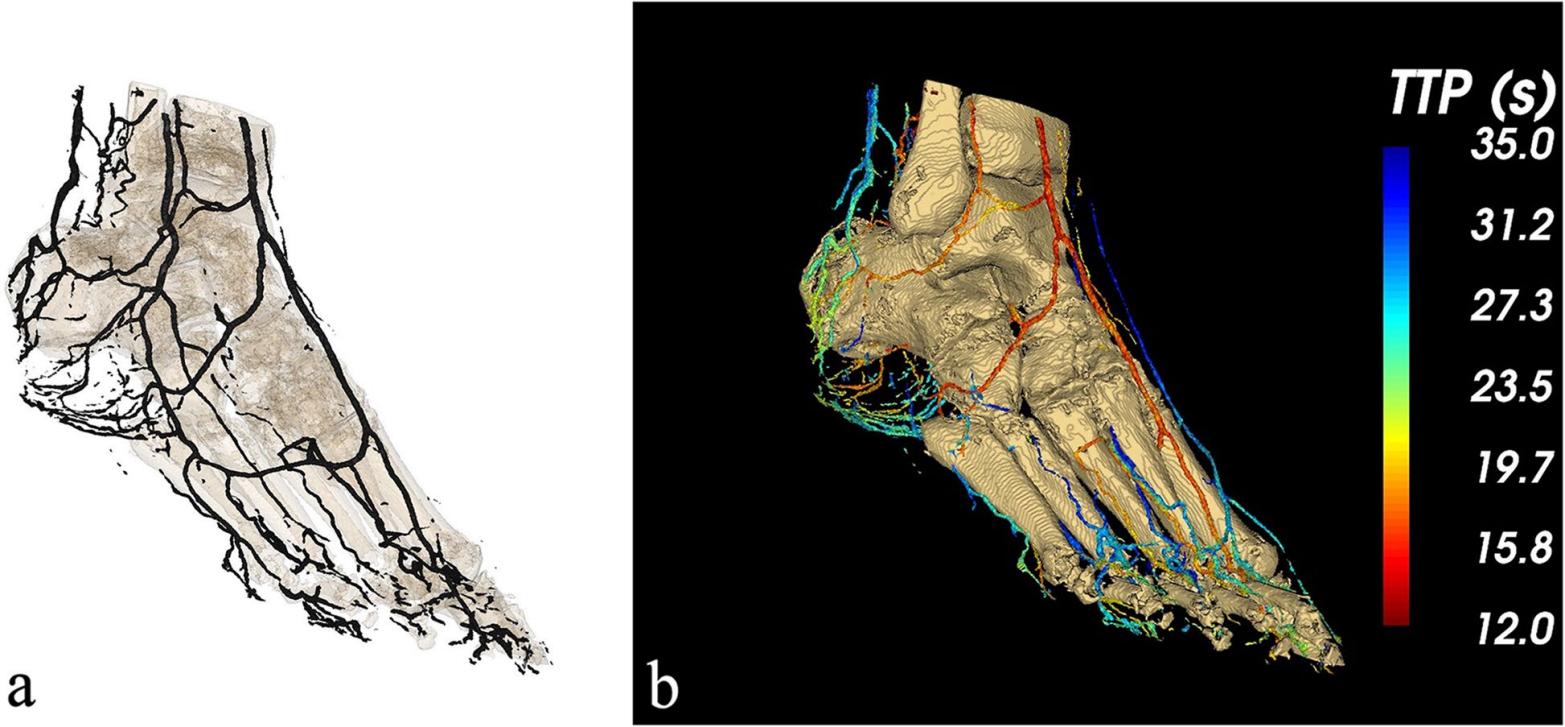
a Rendering of the enhanced arterial segments derived from the 4DCT data (participant number 1) at 18. 9 s after contrast injection. b Volume rendering of the parametric map indicating the contrast arrival (time-to-peak in seconds) for blood vessels by means of color-coding: early arrival of the contrast is depicted in red, late arrival in blue. *4DCT* Four-dimensional computed tomography
